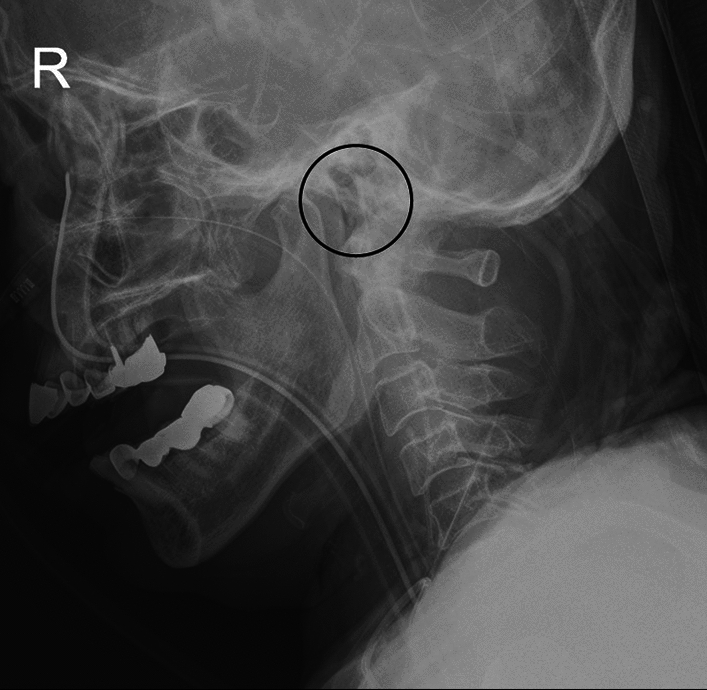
The placement of the catheter tip confirmed using lateral cervical spine X-ray. Lateral cervical spine X-ray documented a correct positioning of the jugular bulb catheter tip (black circle).
